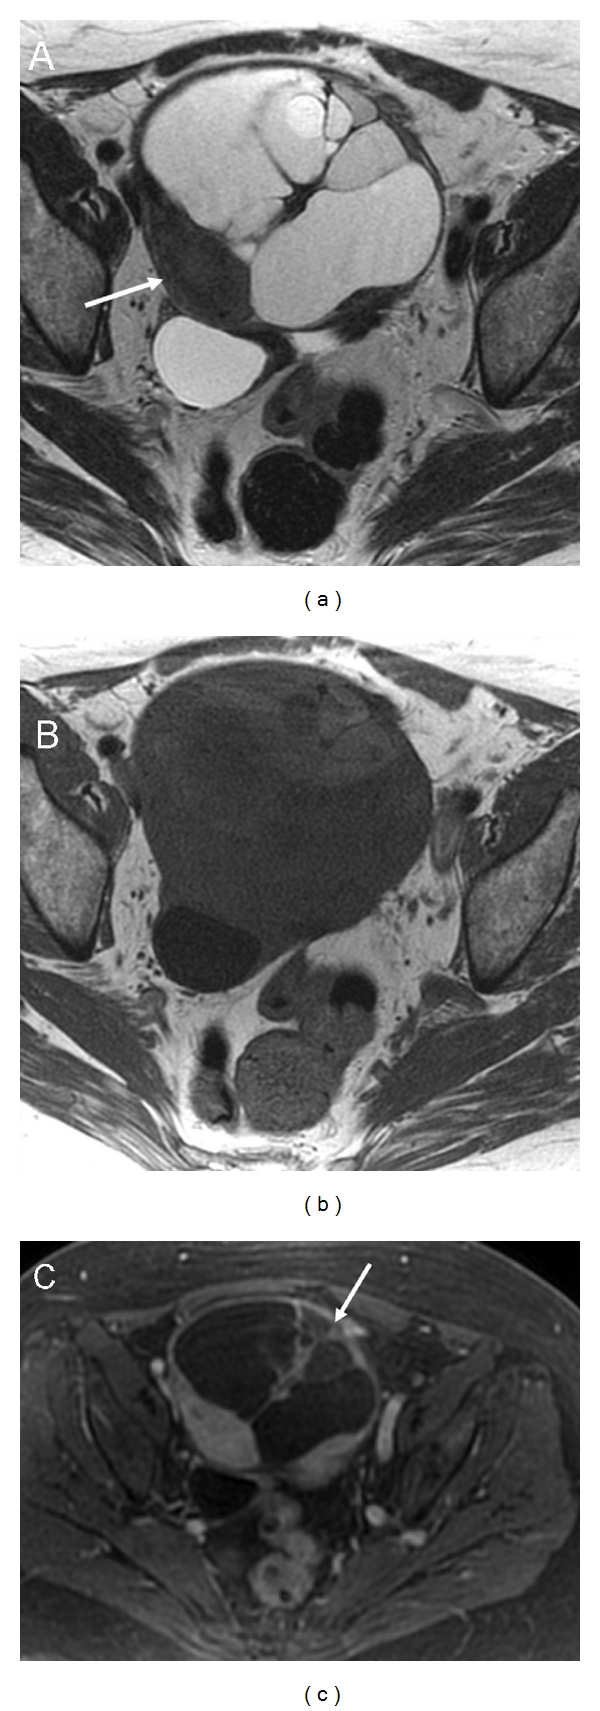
Brenner tumour with mucinous portion. Collision tumour. Axial T2- (a) and T1-weighted (b) images demonstrate an adnexal multilocular cystic mass with thin septations and a solid posterior hypointense portion (a, arrow). The cystic portion presents multiple loculi with different contents characterized by different signal intensity. The septations and the solid portion present an homogeneous and delayed enhancement on 3D fat-saturated T1-weighted Spoiled Gradient Echo images (c, arrow). The interface between the solid and the cystic mass is regular.
